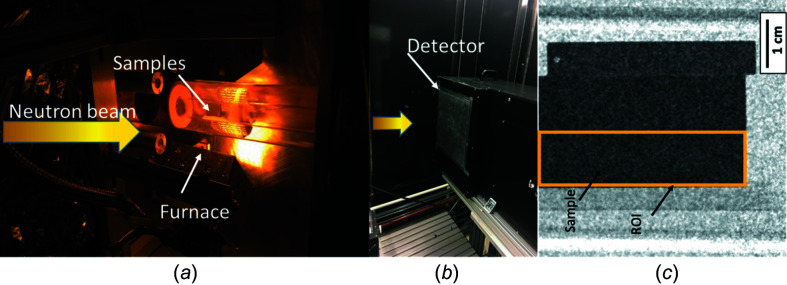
The experimental setup with (*a*) a furnace (placed in front of the detector, which cannot be seen here), with three samples stacked on top one another, and (*b*) a detector (shown without the furnace for better visibility). (*c*) The radiography image of the sample inside the furnace taken at 4.02 Å neutron wavelength [note that there are three samples, but this study focuses only on the sample that is highlighted by an orange rectangle (ROI)].
